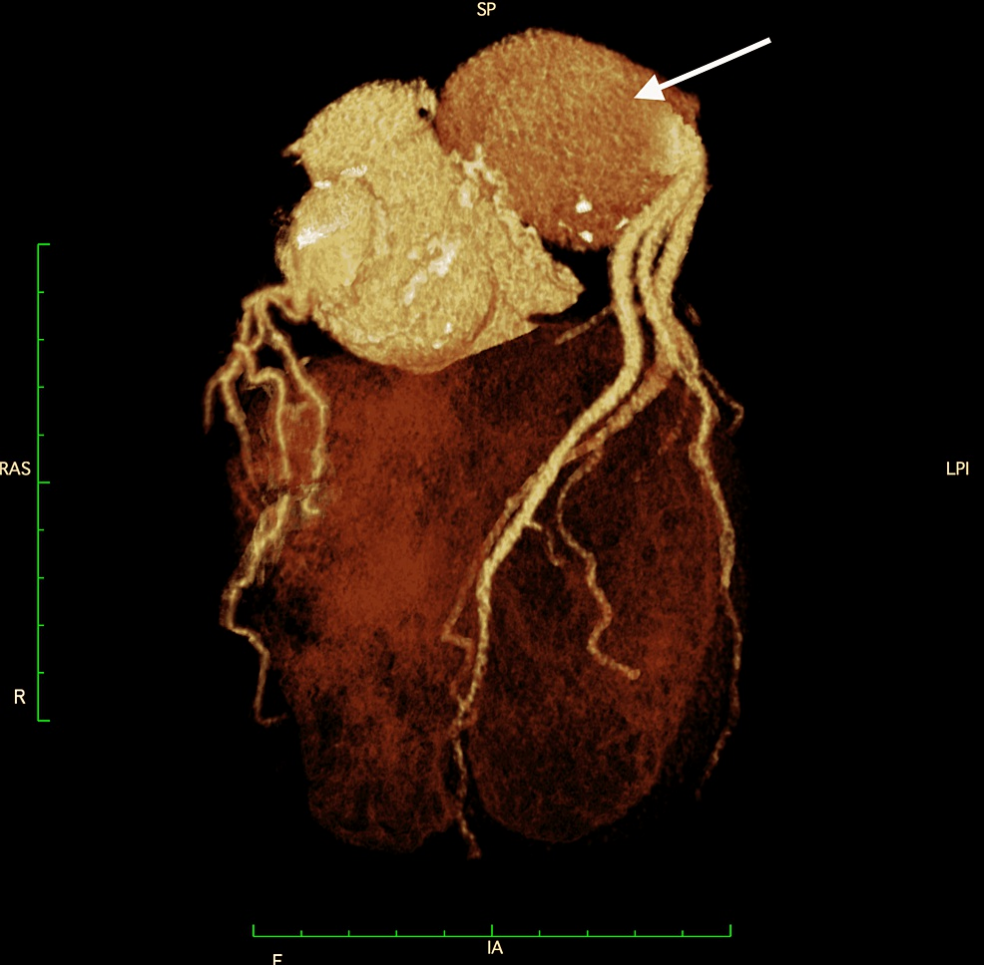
Chest computed tomography before the third surgery. Chest computed tomography before the third surgery. The left coronary button aneurysm enlarged up to 73 mm in diameter, while the right coronary button aneurysm was slightly enlarged.
